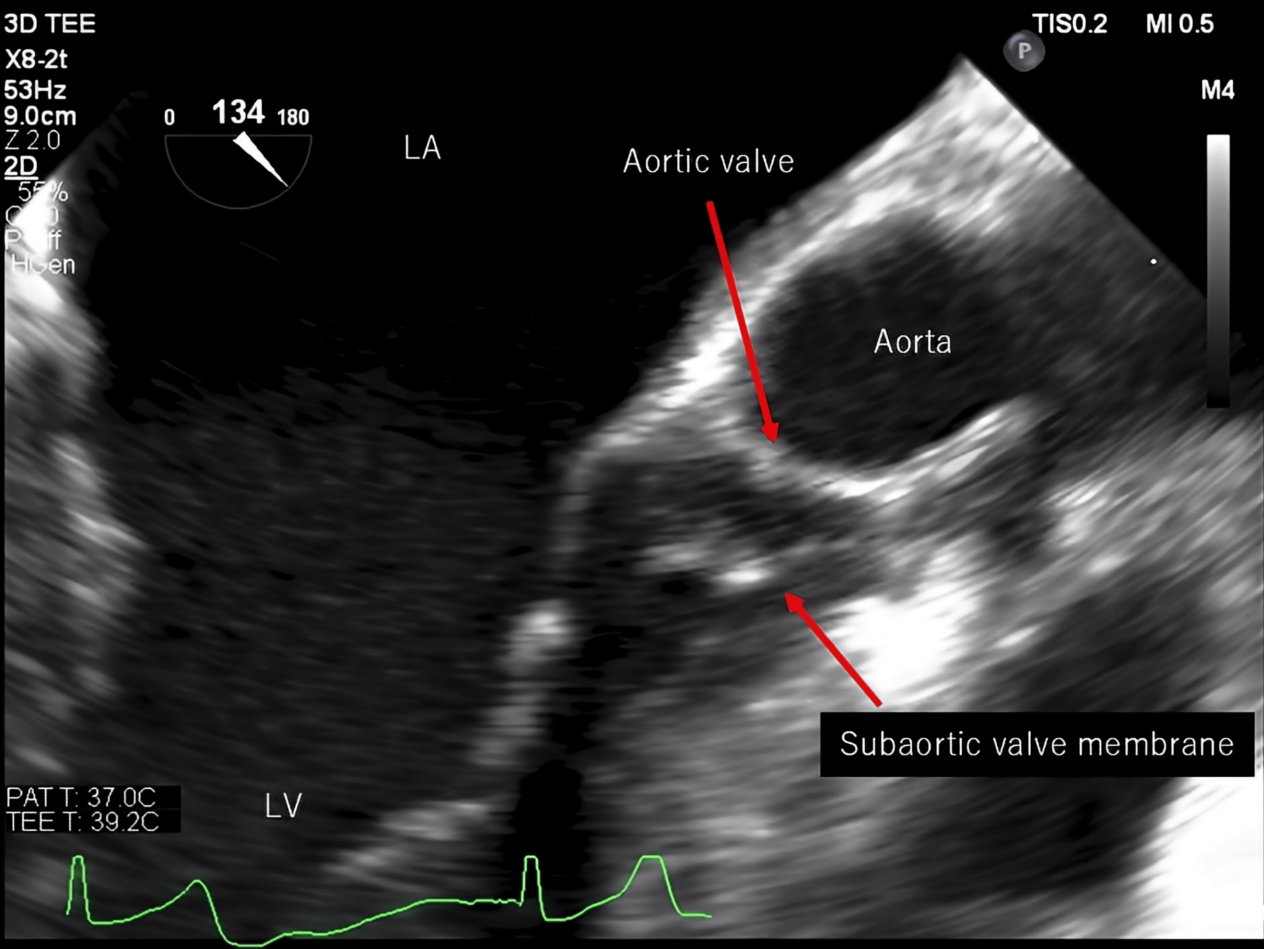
Transesophageal echocardiogram A membrane structure was identified below the aortic valve.
